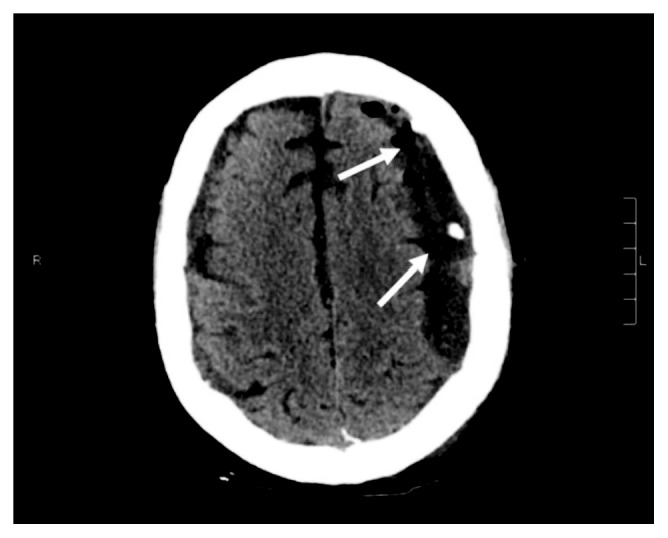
Computed tomography of the head.
